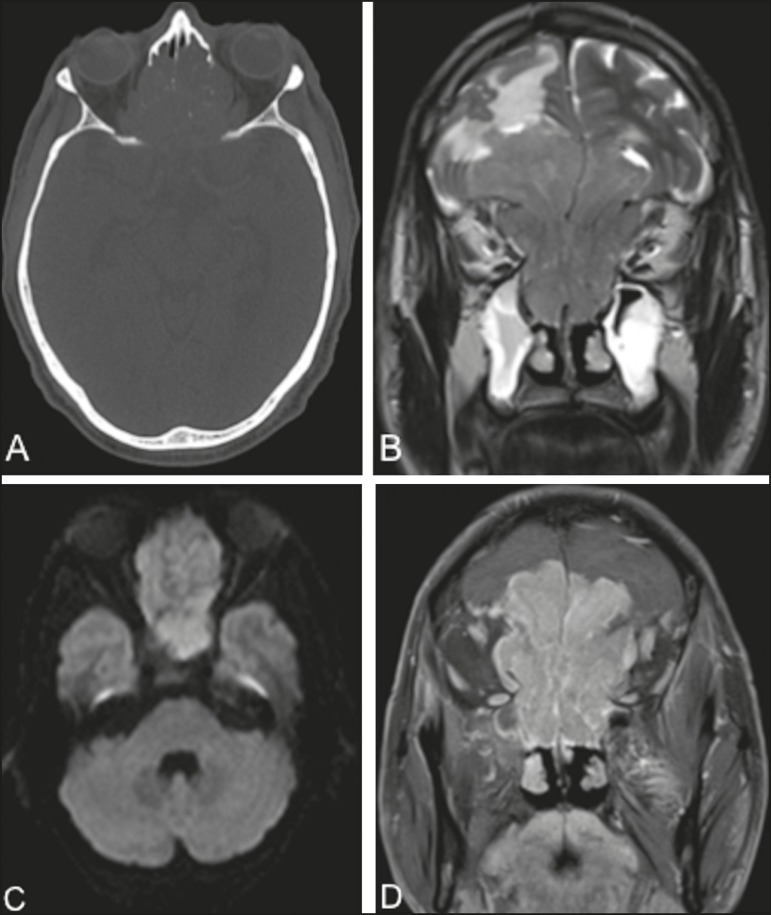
CT of the brain (A), with a bone window, showing an expansile lesion
